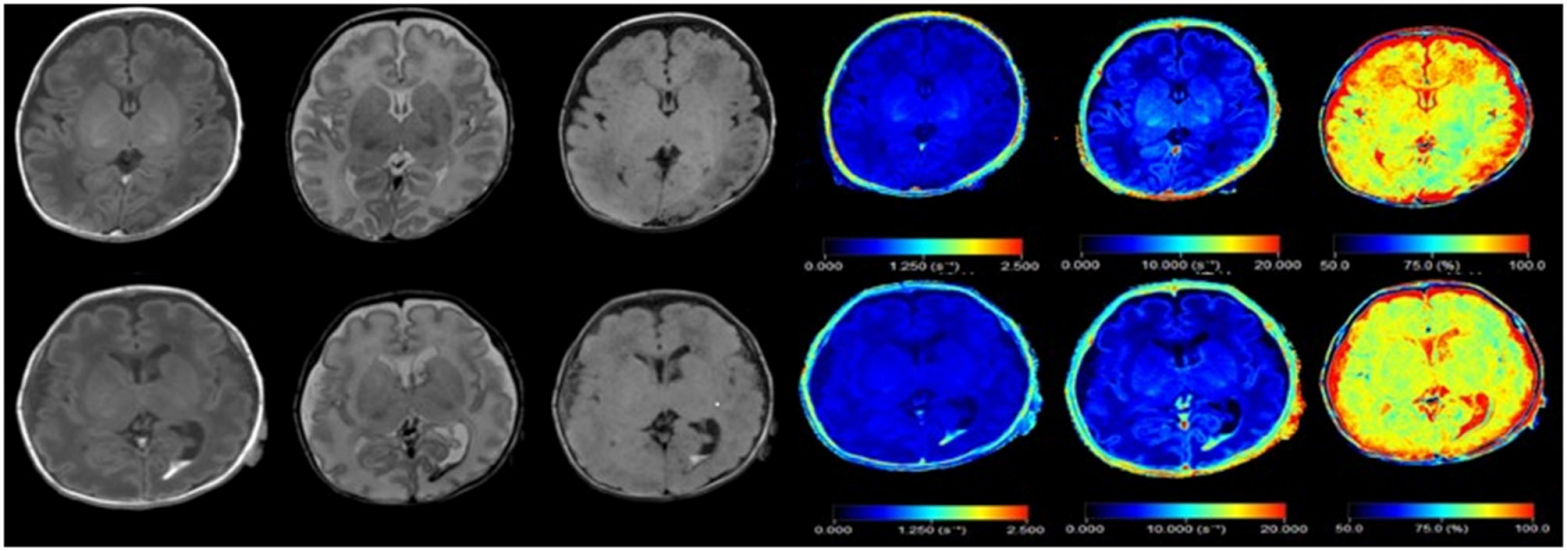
Synthetic images of a normal premature infant, and premature infant with intraventricular hemorrhage. Upper row: Synthetic MRI of normal preterm infants, in order of T1, T2, T2 FLAIR, R1, R2, PD. Bottom row: Synthetic MRI of premature infants with intraventricular hemorrhage, in order of T1, T2, T2 FLAIR, R1, R2, PD. (R1 = 1/T1, R2 = 1/T2).
